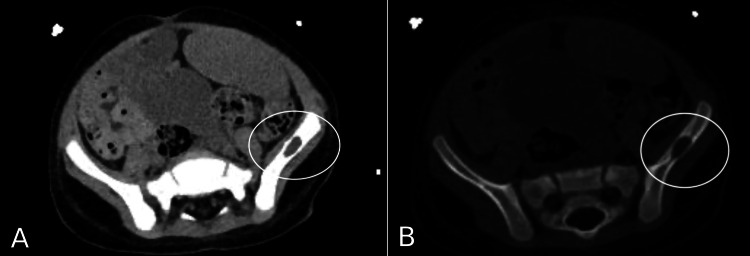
CT scan showing 1.5 cm lytic lesion in the left iliac wing.
